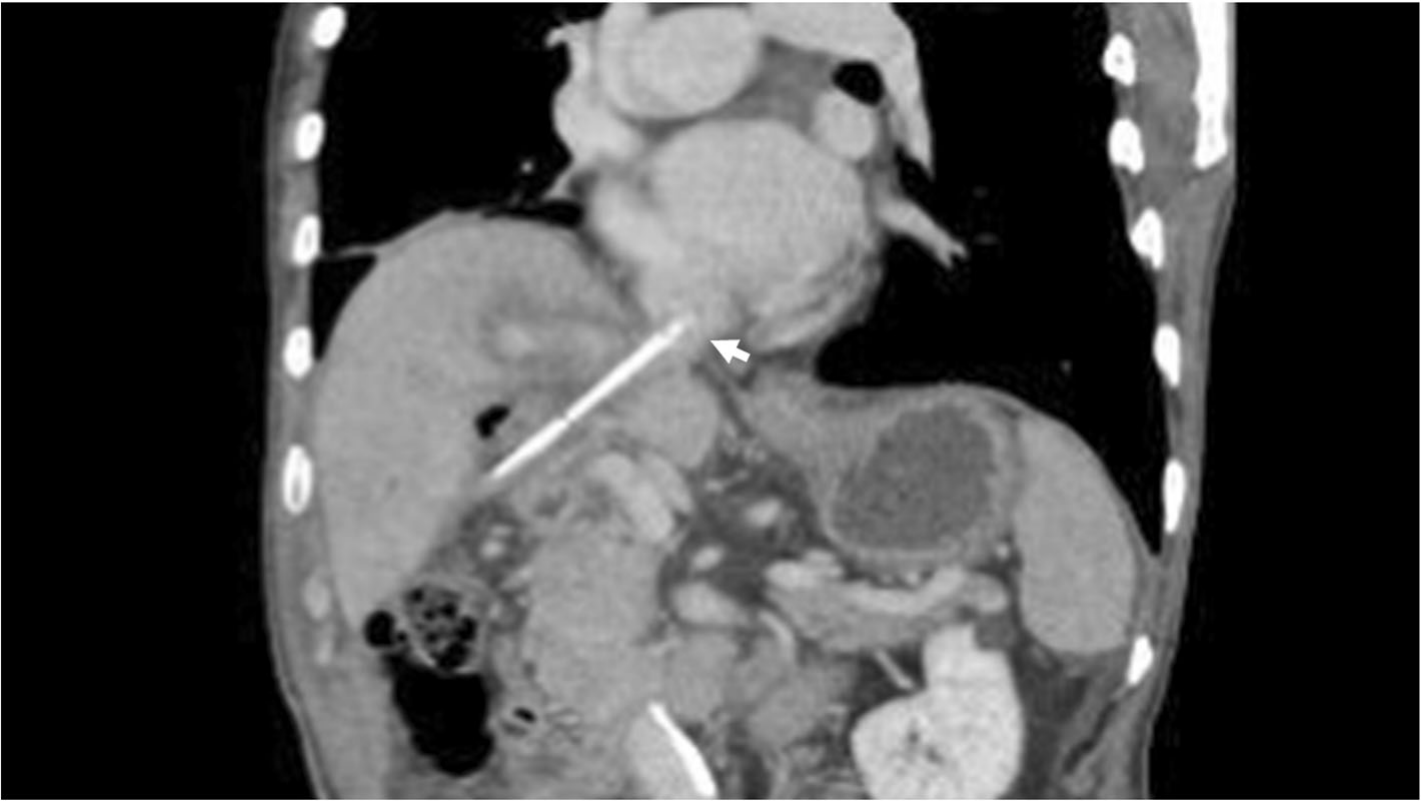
An enhanced abdominal CT scan showed the tip of the drain perforating the IVC and straying into the RA (arrow). *IVC* inferior vena cava, *RA* right atrium
